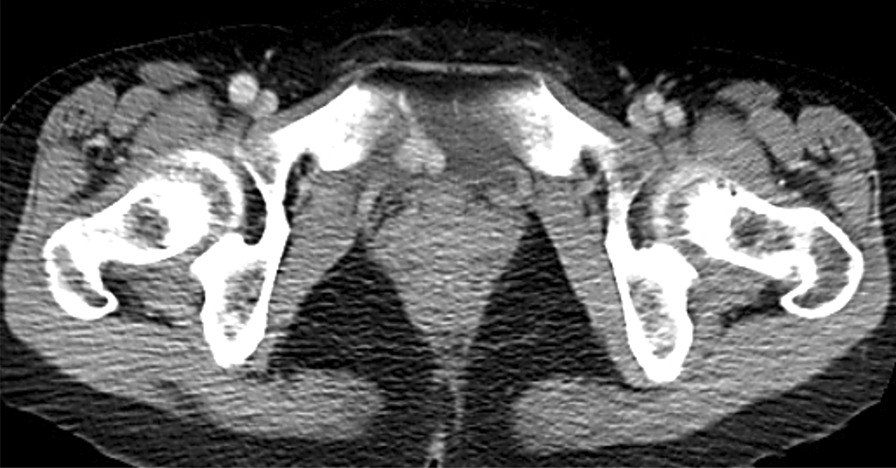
Contrast-enhanced pelvic computed tomographic image showing a well-enhanced intraluminal bladder mass, consisting of components
